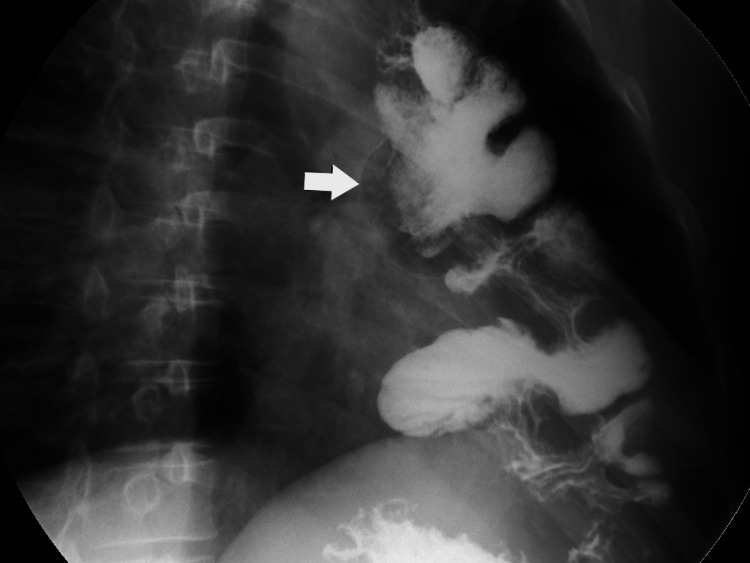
Contrast roentgenography, showing a well-functionating colo-oesohpagoplasty. The white arrow shows the intrathoracic retrosternal colon.
